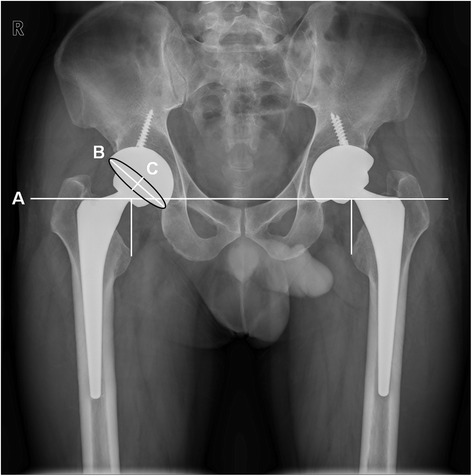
A standardized anteroposterior pelvic radiograph of a 39-year-old male patient with bilateral osteonecrosis of the femoral head at 6 months after simultaneous bilateral total hip arthroplasty showing the radiographic measurement of leg-length discrepancy and acetabular cup orientation. The line A indicates the inter-teardrop line as a reference line. The line B is the long axis and the line C is the short axis of the opening ellipse of the acetabular component, respectively
